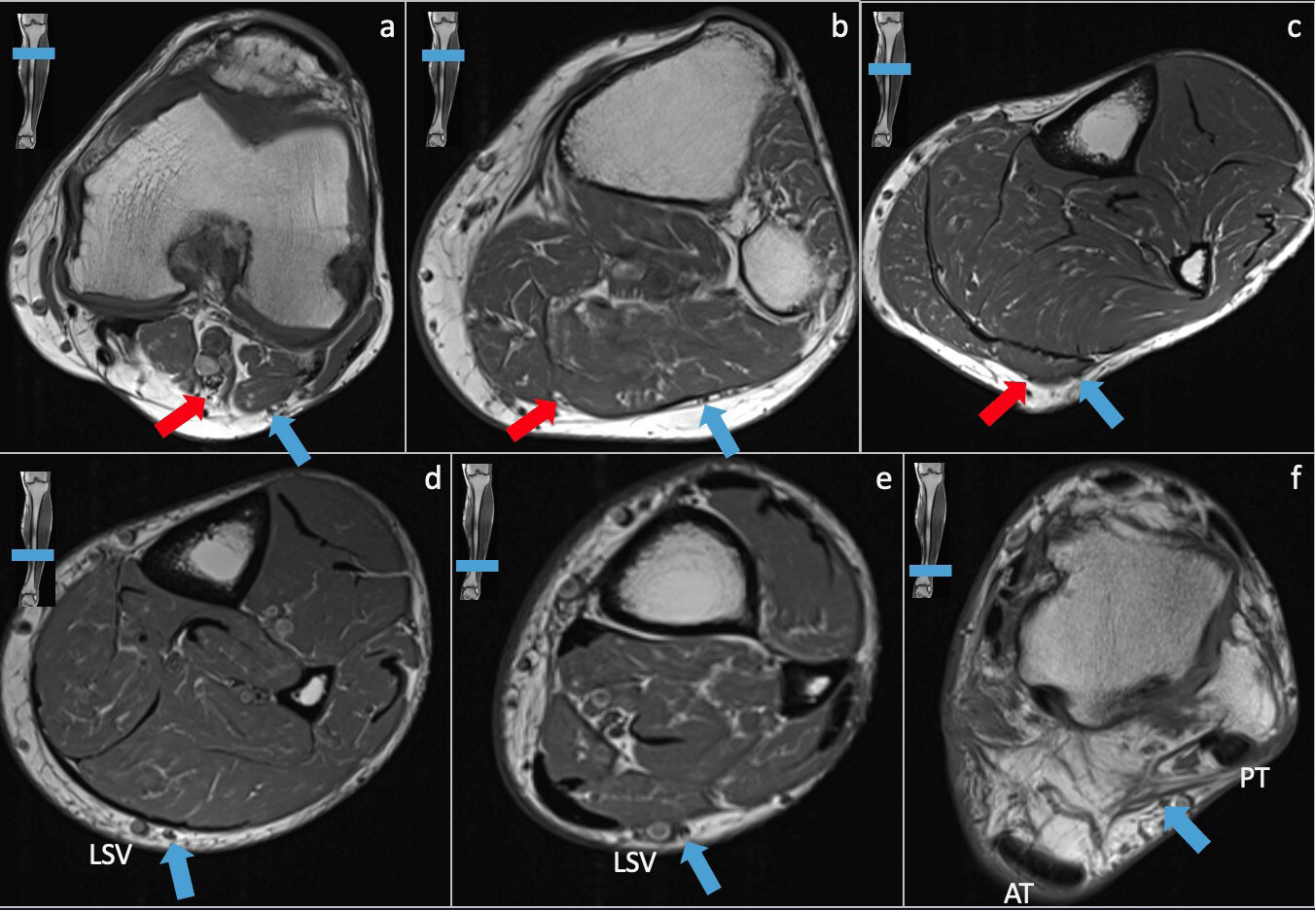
Normal MRI anatomy of the sural nerve. The sural is formed by the fusion
